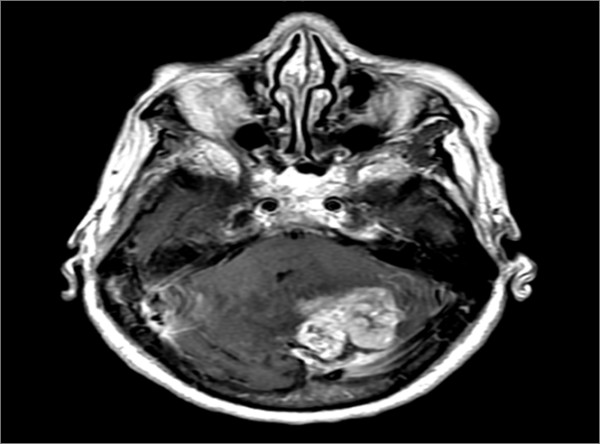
Brain MRI showed hemorrhagic mass involving the medial aspect of the left cerebellum and crossing the midline to the right hemisphere.
